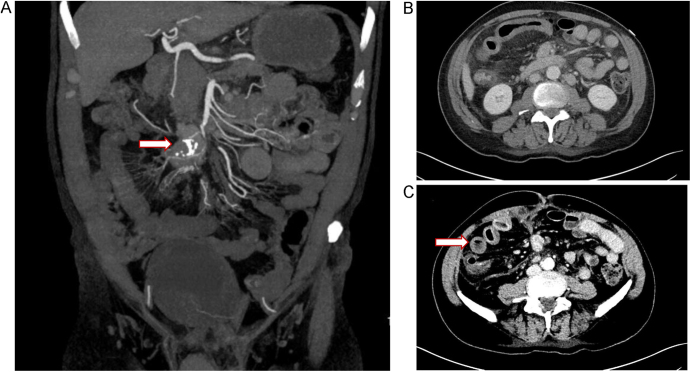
CT scan of patient 3. (A) Mesenteric mass causing stenosis of the distal branches of the SMA. (B) Post-PRRT: long segment of ileum with dilation and circumferential wall thickening. (C) Mucosal hyperenhancement indicating ischemia.
